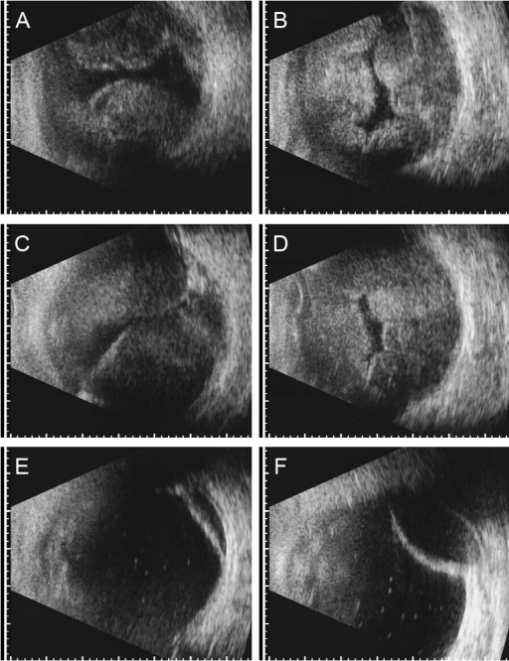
Case 1, transverse B-scan ultrasonography of the left eye. A, B Six days preoperatively. Massive suprachoroidal hemorrhage with circular kissing choroids. Visual acuity was hand motion. A from inferior. B from temporal. C, D Two days preoperatively. The ultrasonic findings deteriorated, the vitreous cavity was increasingly constricted. Visual acuity was light perception. C from inferior. D from temporal. E, F 14 months postoperatively, six days after removal of silicone oil. The choroid was widely reattached, a limited choroidal detachment persisted in the temporal and nasal periphery. Visual acuity was 20/80. E from nasal. F from temporal
